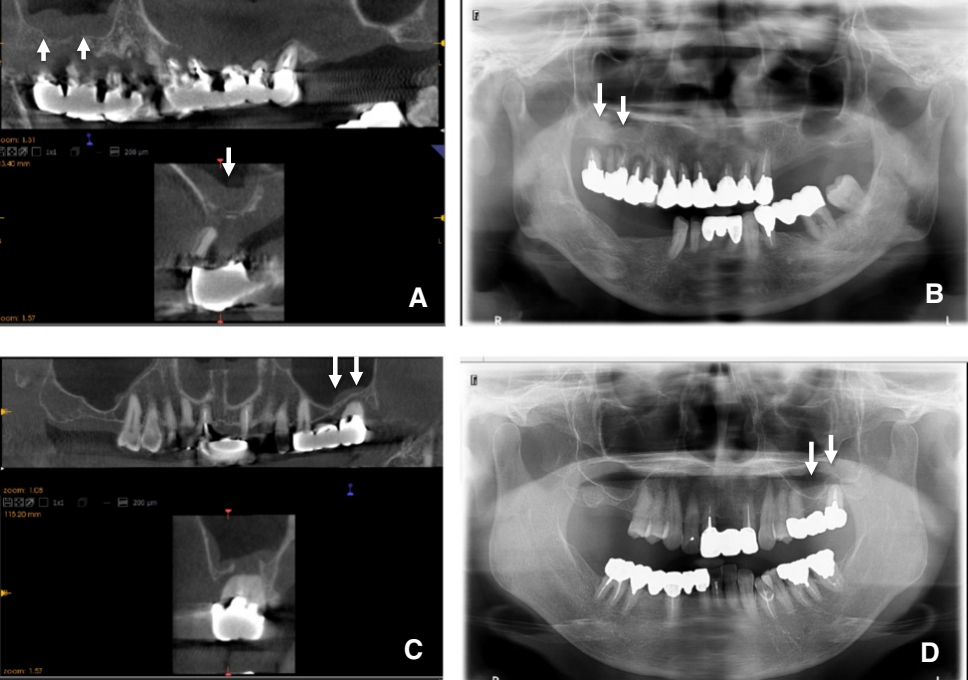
Images of SOLs in the maxillary sinus. The CBCT (A) and panoramic radiograph (B) of a 63-year-old female with an SOL with a major axis length >4 mm undergoing preoperative planning for implantation of a left maxillary molar. The CBCT (C) and panoramic radiograph (D) of a 70-year-old female with an SOL with a major axis length <4 mm undergoing preoperative planning for implantation of a left maxillary molar. The SOL is clearly visualized on CBCT (A and C). However, the SOL with a major axis length >4 mm is visualized on panoramic radiographs (B), but that < 4 mm is not (D).
